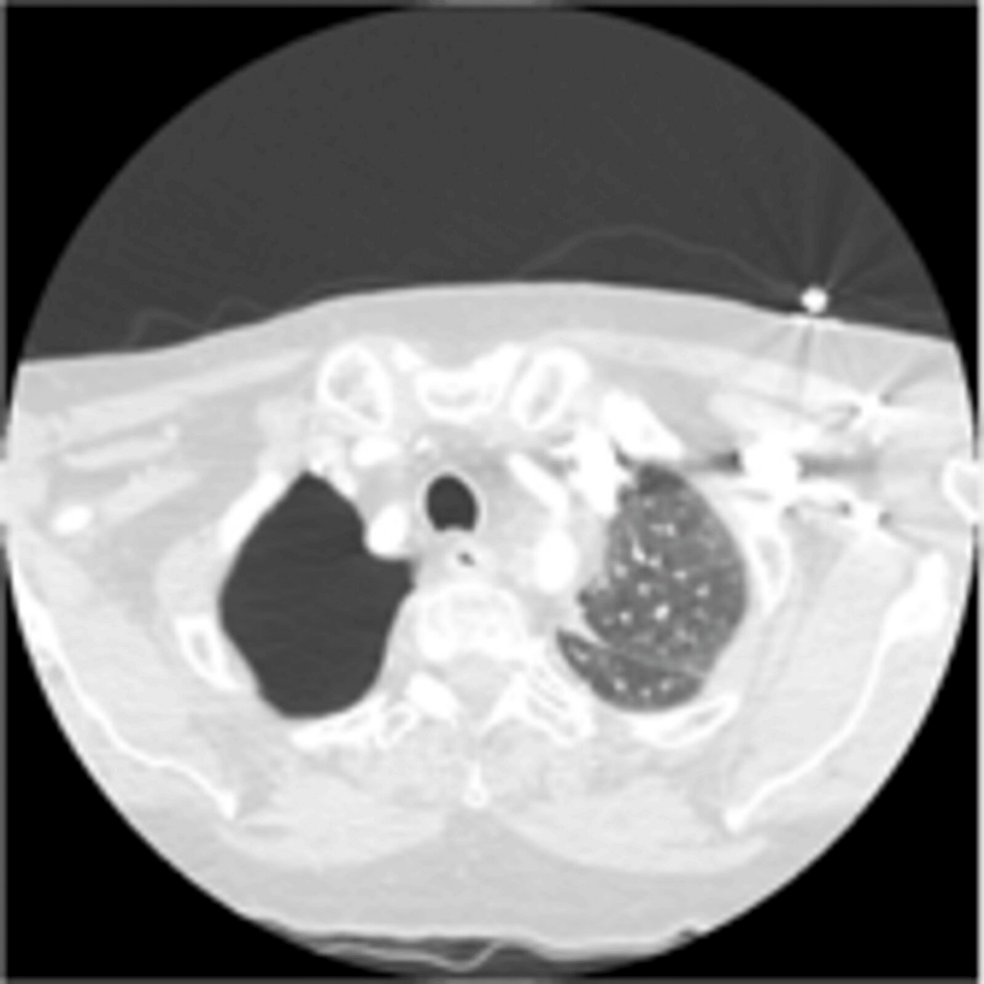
Lower cuts of the computed tomography angiography(CTA) scan of the neck showing a right apical pneumothorax
